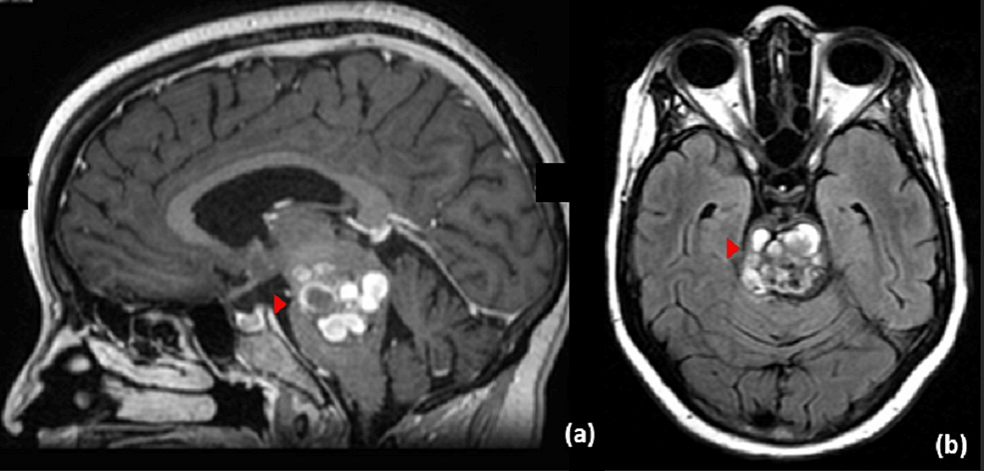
Pre-operative magnetic resonance imaging Pre-operative sagittal (a) and axial (b) cut of magnetic resonance imaging showing a mass lesion occupying the pontine isthmus with variable signal intensities measuring about 21 x 17 mm that is consistent with cavernous hemangioma causing local mass effect.
